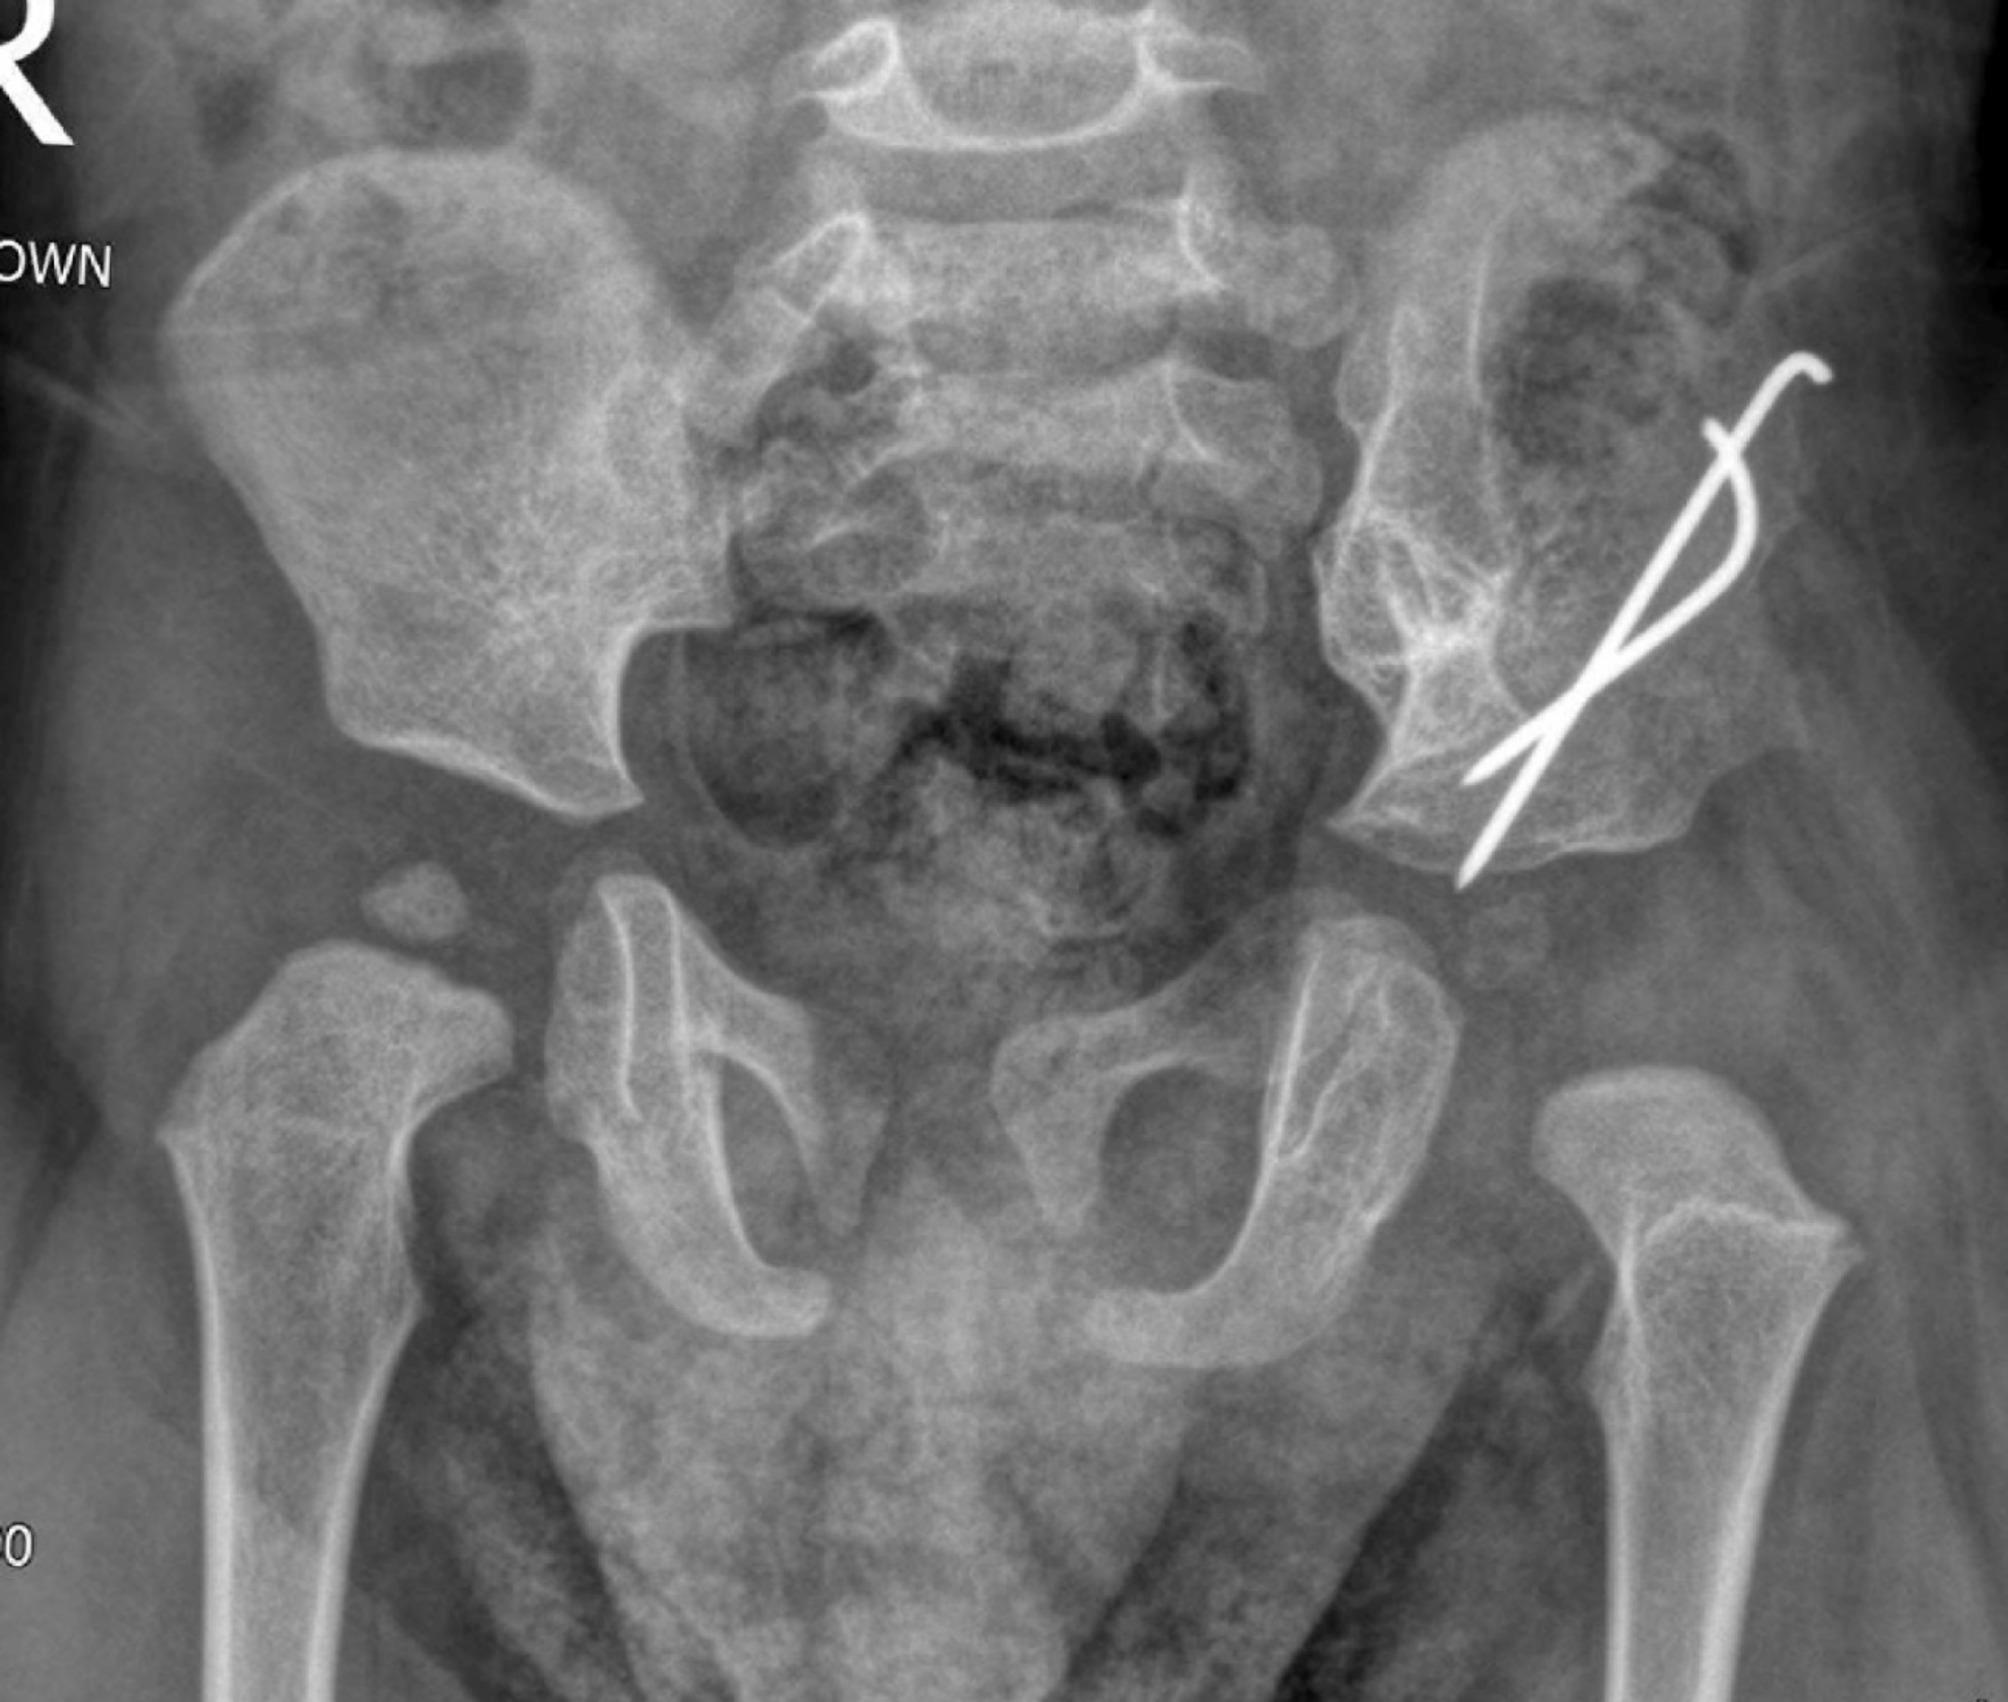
Anteroposterior pelvic radiograph 18 months after initial resection and 4 months after open reduction of the left hip, showing reduction of the hip
